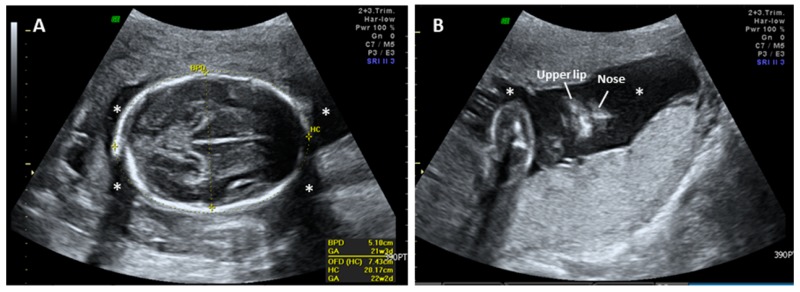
Prenatal ultrasound images of the fetus with renal tubular dysgenesis (RTD) at 22^+2^ weeks of gestational age (wGA) showing structural normality without oligohydramnios. (A) Axial and (B) coronal view of the fetus. The black space surrounding the fetus is amniotic fluid (stars). BPD: biparietal diameter, HC: head circumference.
